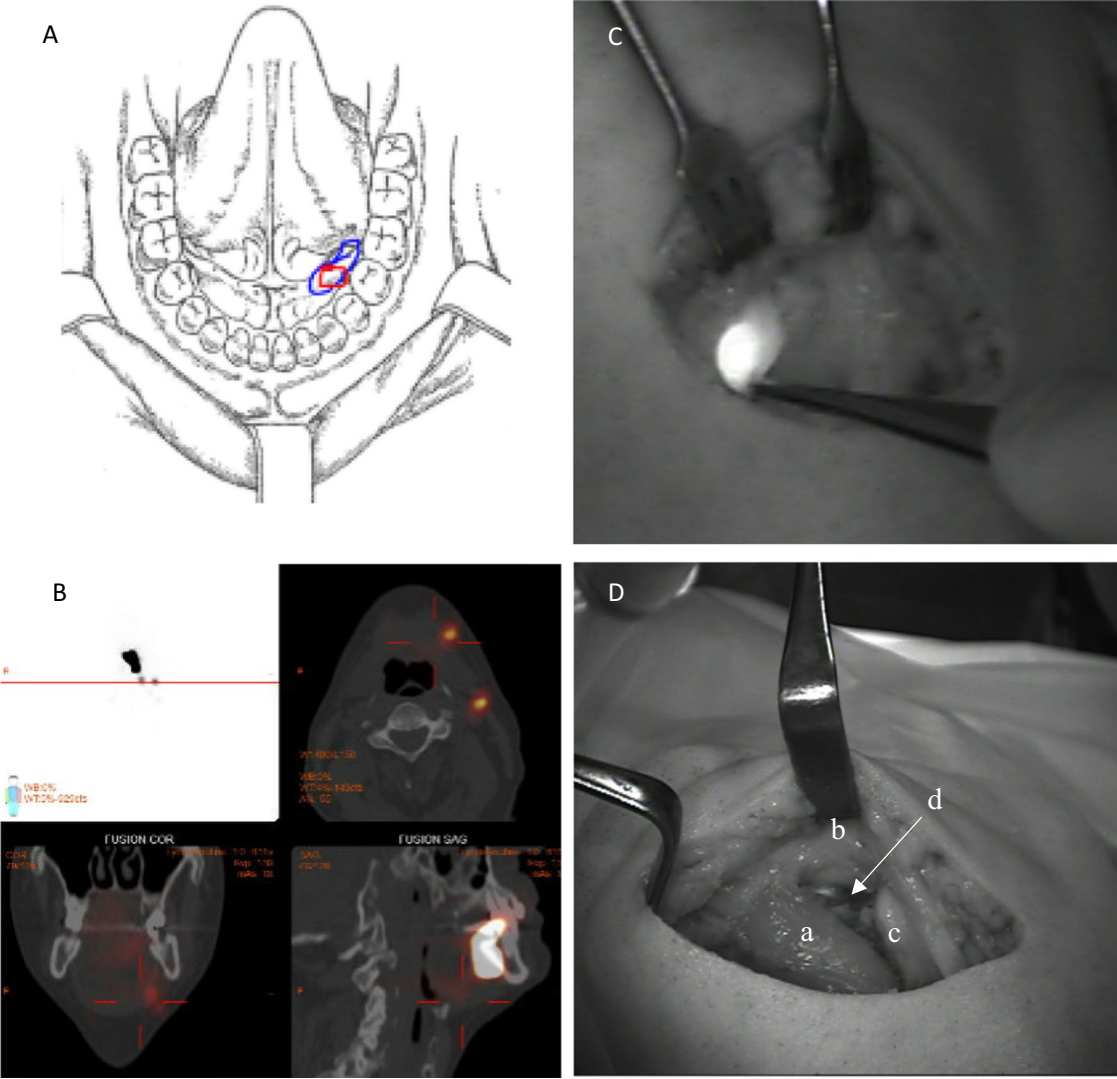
Optical imaging with the Fluobeam 800 system. An example of SNB staging of a cT1N0M0 lesion in the left FOM without midline involvement (A). SPECT/CT detected 2 SNs in the ipsilateral level 1b and 2a (B). Intraoperative optical imaging of the SN harvested in level 1b (C). The SN was directly visualized by optical imaging just below the edge of the mandible where navigation with the handheld gamma probe was impaired. The surgical field in the anterior level 1b after completed super-selective ND (D): Anterior belly of the digastric muscle (a), lower edge of the mandible (b), anterior portion of the submandibular gland (c), the location of the SN (d)
